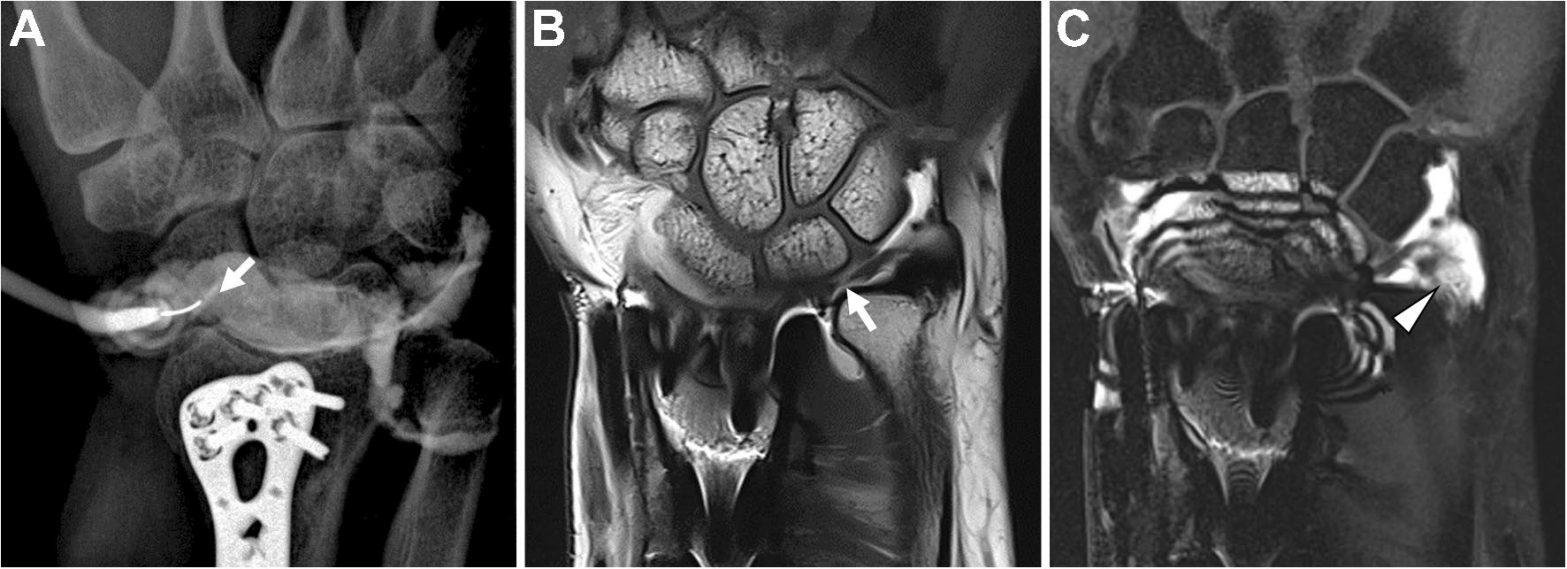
Postoperative wrist imaging. Thirty-eight-year-old man with wrist pain following radius fracture and surgery. A Fluoroscopic-guided direct arthrogram image shows flow of contrast from the radiocarpal joint into the distal radioulnar joint. Coronal T1-weighted 1.5 T MR arthrogram images without (B) and with (C) fat-suppression show the triangular fibrocartilage complex tear involving the central disc (arrow) and ulnar attachments (arrowhead), but the scapholunate interosseous ligament is obscured by artifact. The integrity of the scapholunate and lunotriquetral interosseous ligaments can be assumed by the lack of contrast extension into the midcarpal compartment
